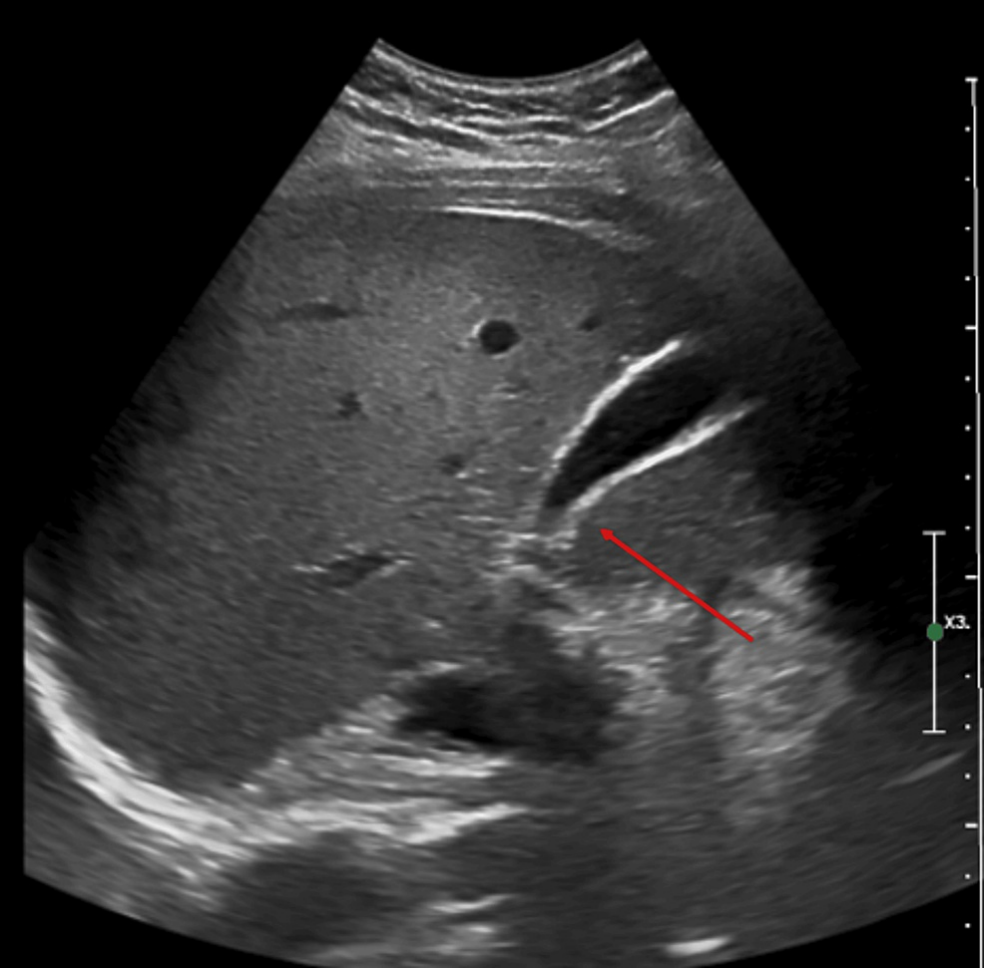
Ultrasonography shows normal gallbladder with no CBD dilation. CBD: common bile duct
